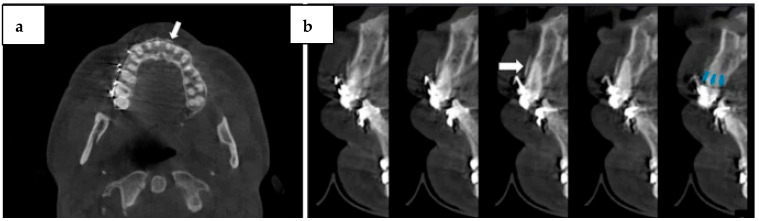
(a) Longitudinally displaced cortical plate fracture (arrow) is visible in the axial CBCT image. (b) Alveolar fracture (white arrow) and radiolucent artifact from metal brackets mimicking a crown fracture (blue arrows) are seen in cross-sectional CBCT images. Published with permission from [34]. Copyright 2021, Elsevier.
